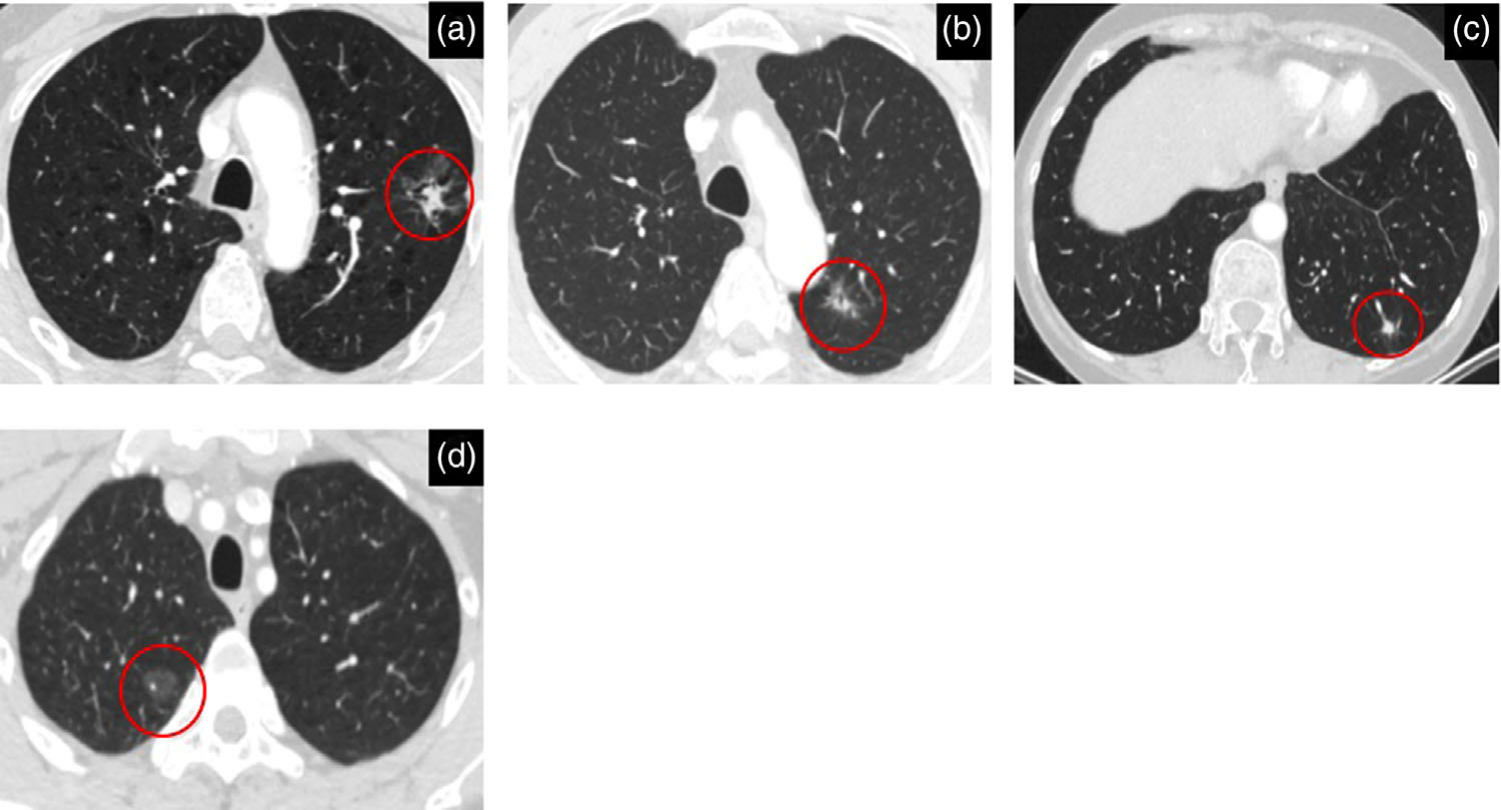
Computed tomography images showing solid and subsolid lung nodules: (a) mixed ground‐glass malignant (invasive adenocarcinoma) nodule peripherally situated in the left upper lobe measuring 25 mm max. diameter. (b) Mixed ground‐glass malignant (invasive adenocarcinoma) nodule situated peripherally in the left upper lobe measuring 14 mm max. diameter. (c) Solid benign nodule is situated peripherally in the left lower lobe measuring 248 mm^3^. (d) Pure ground‐glass malignant (adenocarcinoma in situ) nodule situated peripherally in the right upper lobe measuring 14 mm max. diameter.
